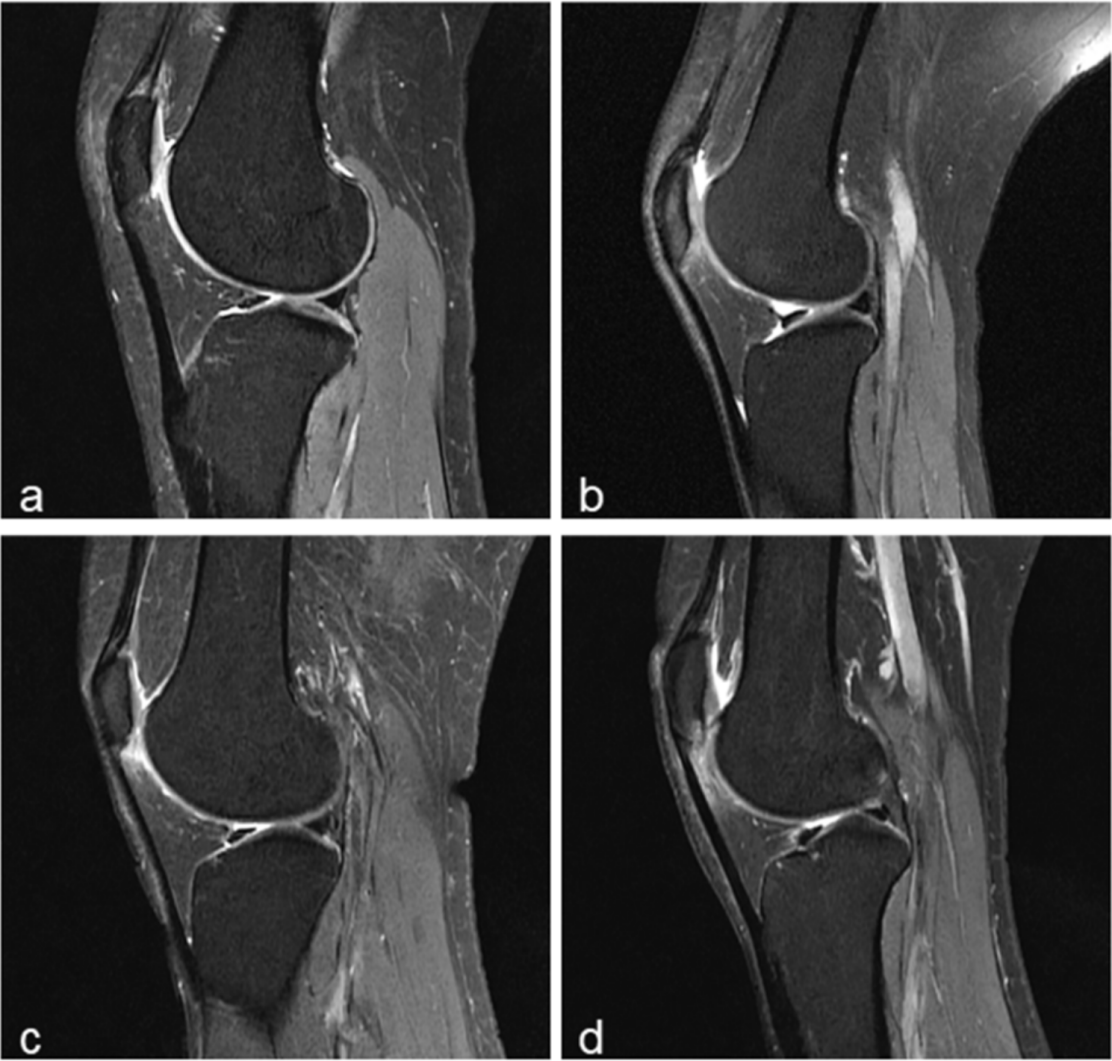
Superolateral Hoffa fat pad edema (SHFPE). Sagittal magnetic resonance imaging of the knee shows the SHFPE scoring system for a normal, b mild or grade 1, c moderate or grade 2, and d severe or grade 3 SHFPE.
